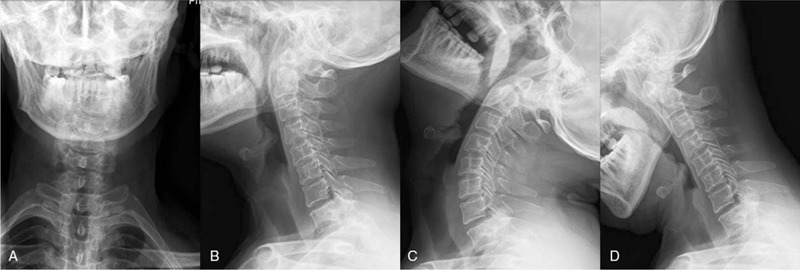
Cervical radiography plains. (A) anteroposterior and (B) lateral radiographs showed abnormal morphology of the C2 vertebral body; (C, D) dynamic radiographs revealed poor stability of C1-C2 segments.
